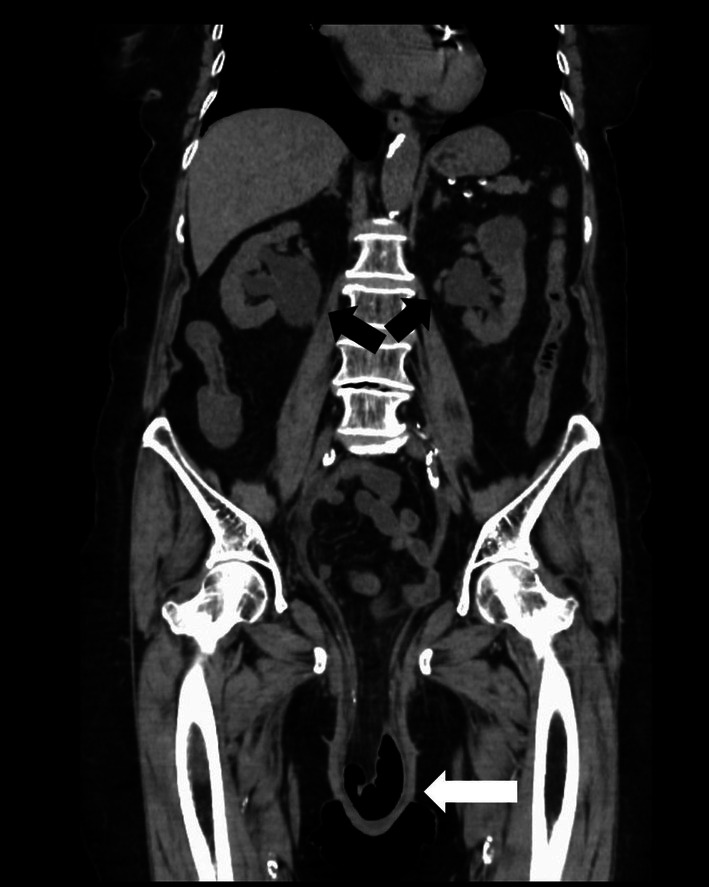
CT findings. Abdominal CT showed severe pelvic organ prolapse (white arrow) and bilateral hydronephrosis (black arrows).
